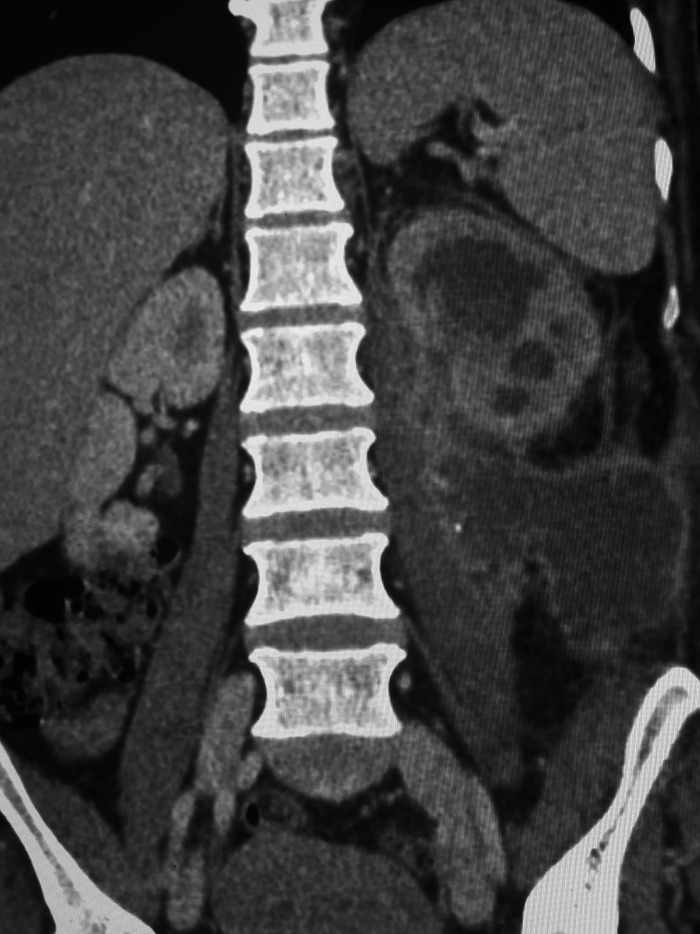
Coronal section of CT of the abdomen showing large retroperitoneal collection around the left kidney infiltrating the ipsilateral psoas muscle.
